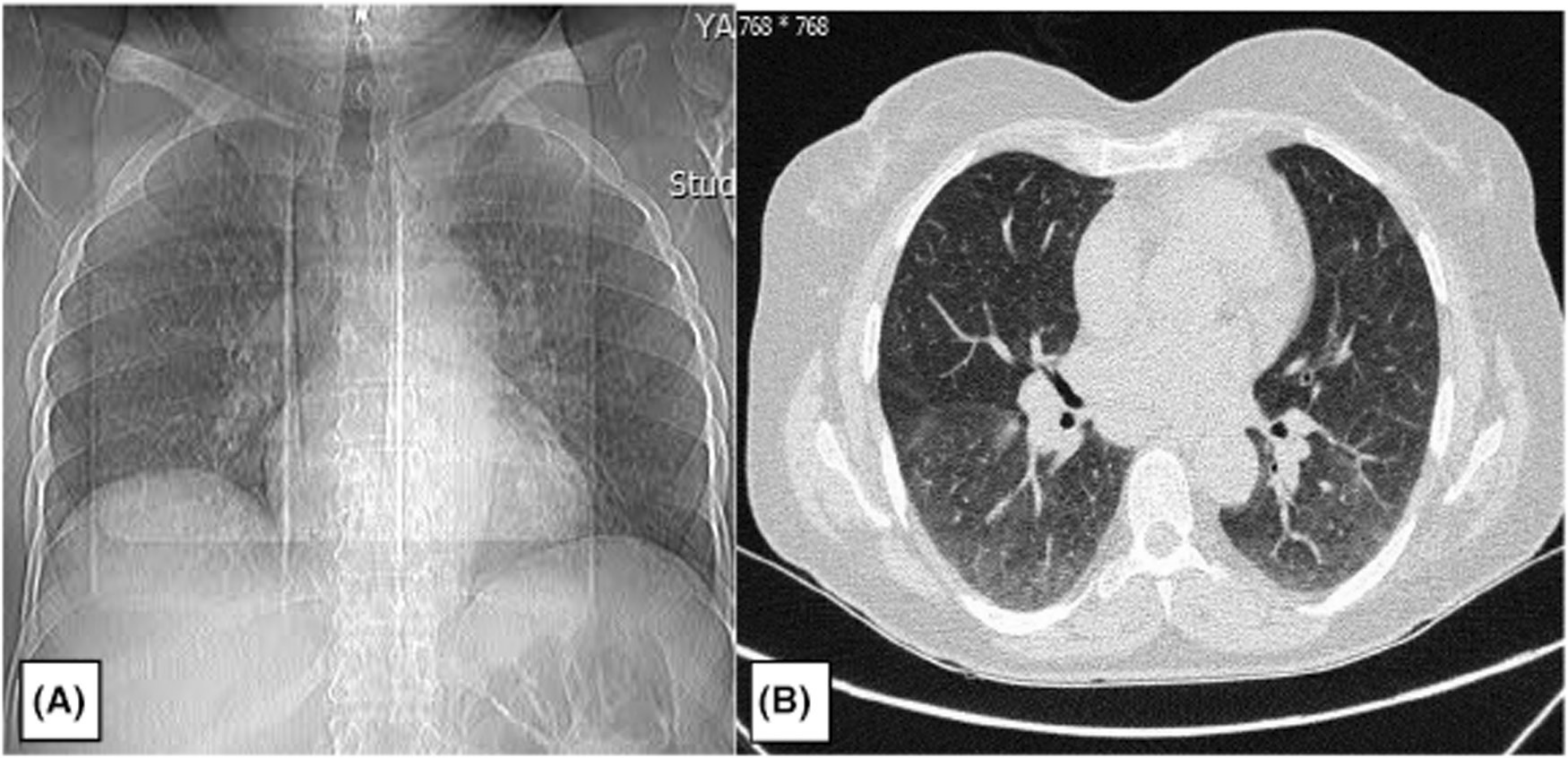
Chest X‐ray (A) and computed tomography scan (B) of a 55‐year‐old female patient with sarcoidosis, demonstrating bilateral hilar lymphadenopathy accompanied by faint ground‐glass opacities
